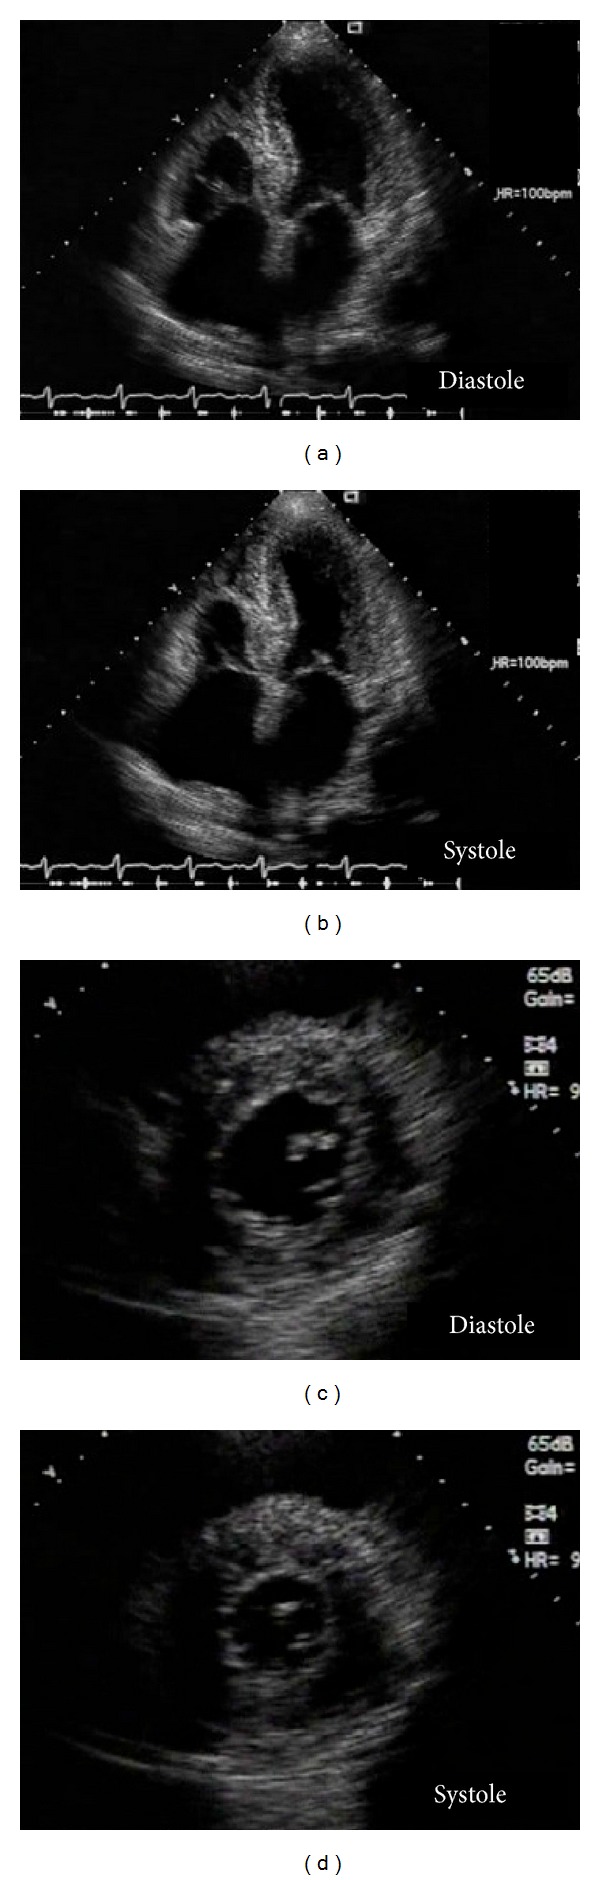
Transthoracic echocardiography 4-chamber (a and b) and short axis (c and d) views show concentric left ventricular (LV) hypertrophy, with a sparkling and granular myocardial texture; right ventricular hypertrophy; and bilateral atrial dilatation. The LV ejection fraction is preserved at 53%, and the mass significantly increased to 256.6 g.
